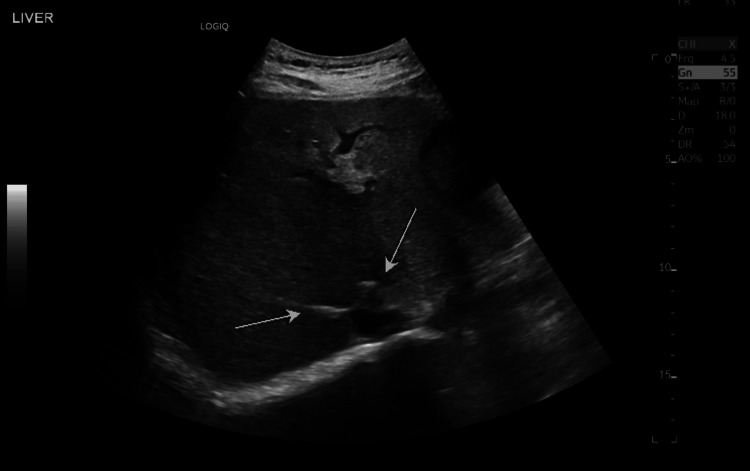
An ultrasound hepatic vein doppler revealed attenuated hepatic veins (indicated by the white arrows)
